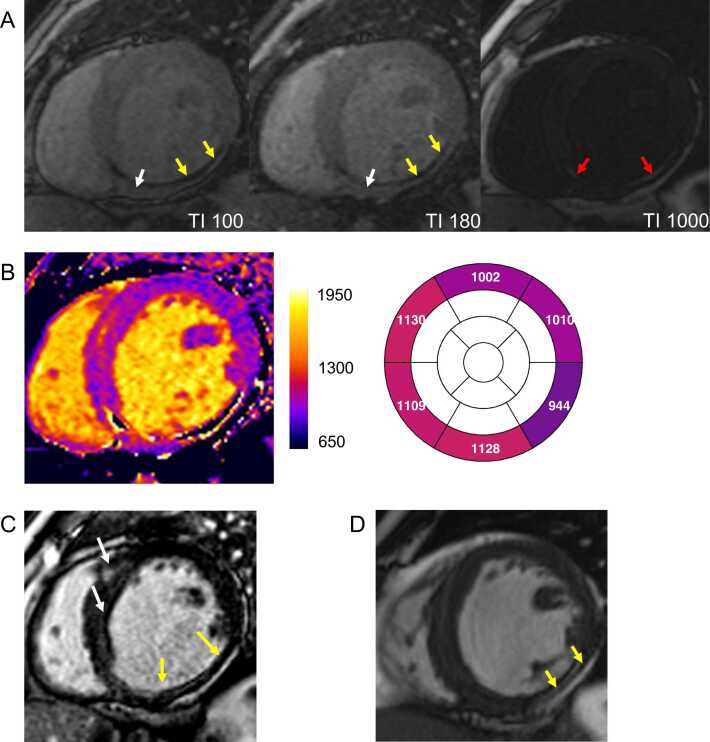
Lipomatous metaplasia in a patient with chronic myocardial infarction. (A) T1-weighted source images show the presence of different tissue types (white, yellow, and red arrows) in the basal inferior wall, reflected by different signal behavior following inversion. (B) Native T1 mapping reveals elevated T1 values in the basal septal and inferior segments (normal 2SD range 905–1037 ms), consistent with chronic myocardial infarction (C, yellow arrows), and focal non-ischemic fibrosis (C, white arrows) as demonstrated on LGE images. In contrast, the basal inferolateral segment shows low-normal T1 values despite evidence of chronic myocardial infarction on LGE images. This discrepancy is likely due to lipomatous metaplasia, characterized by shorter T1 values and visible as intramyocardial hyperintensity on bSSFP cine images (D, yellow arrows). It is crucial to differentiate this pathology from artifacts, as these mapping images provide valuable diagnostic information and should not be excluded from analysis. *bSSFP* balanced steady-state free precession, *LGE* late gadolinium enhancement, *TI* inversion time
